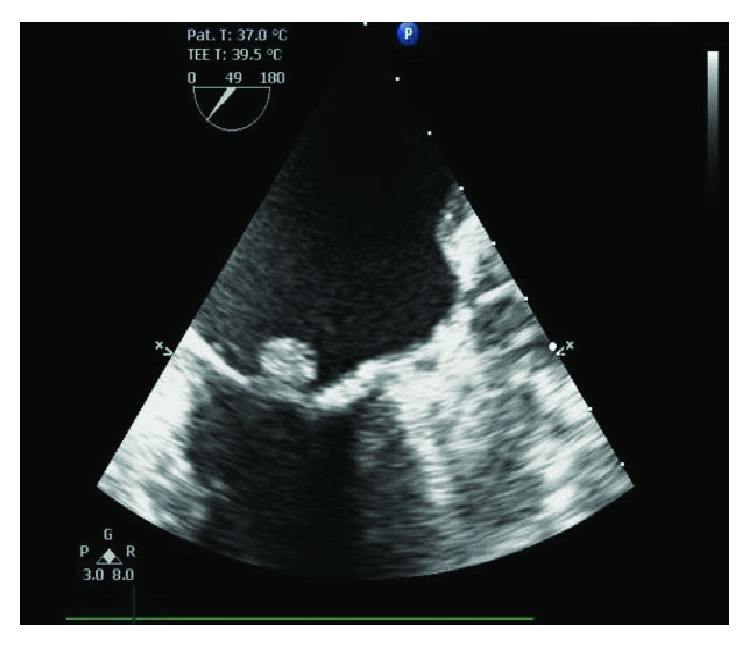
Echocardiogram imaging of a globular mass attached to the mitral valve of heterogeneous echogenicity.
